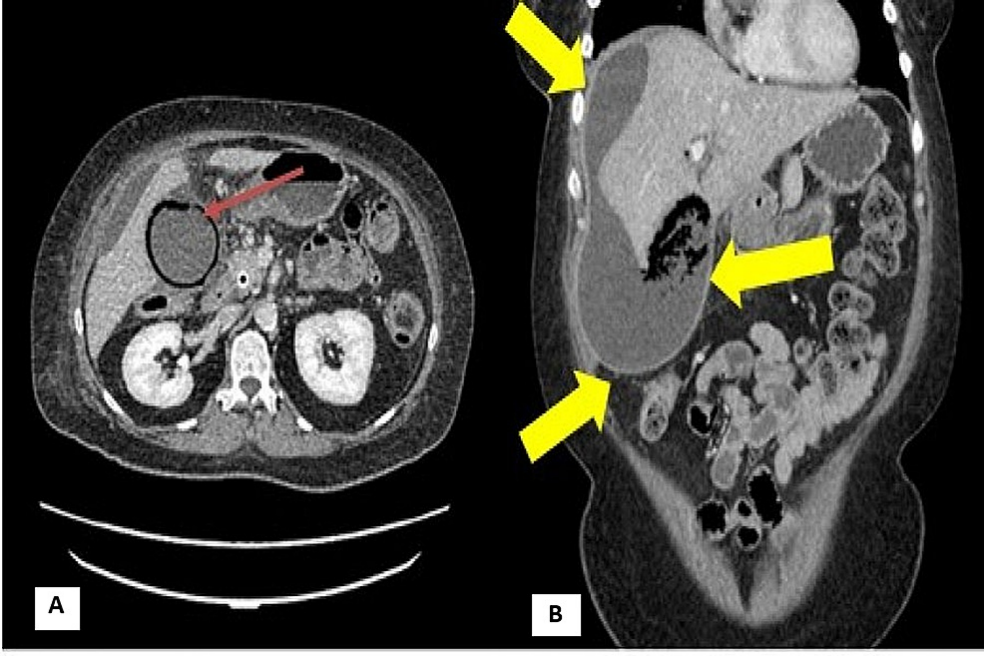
(A) Axial CT of the abdomen with portal venous contrast at the second presentation, showing emphysematous cholecystitis demonstrated by circumferential gas within the gallbladder wall (red arrow). (B) Coronal CT of the abdomen with portal venous contrast, showing the perihepatic collection (yellow arrows) secondary to gallbladder perforation as a complication of cholecystitis. CT, Computed tomography.
